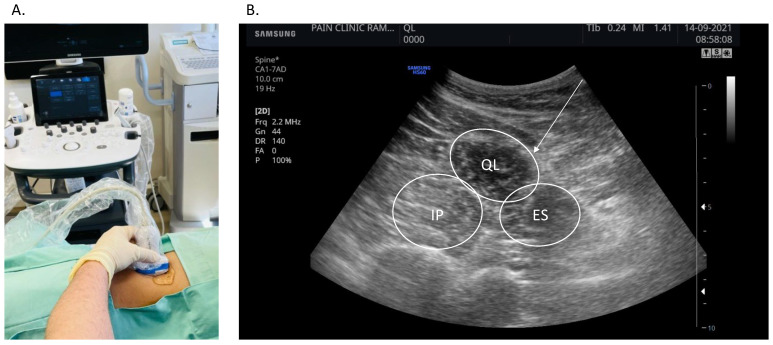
Ultrasound imaging and needling of the quadratus lumborum muscle. (A). Experimental setup demonstrating patient positioning in the lateral decubitus position and transducer placement. (B). A short-axis view of the quadratus lumborum (QL), iliopsoas (IP) and erector spinae (ES) in a healthy individual. The needle trajectory is highlighted. For clarity purposes a short-axis view is presented here; however, needling of the muscle was performed in long-axis.
